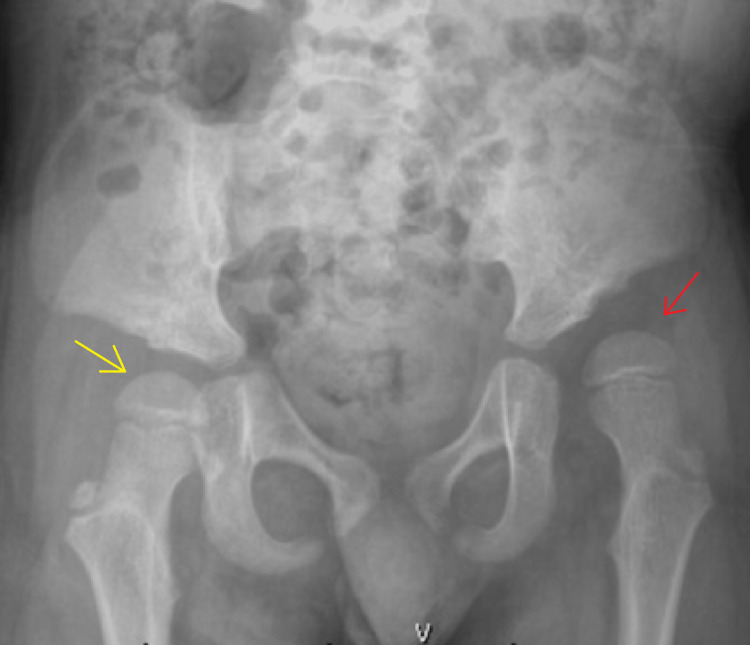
Preoperative AP radiograph of the pelvis demonstrating right sided hip subluxation with Reimer’s migration index of approximately 50% (yellow arrow) and approaching dislocation on the left (red arrow) with bilateral acetabular dysplasia.
